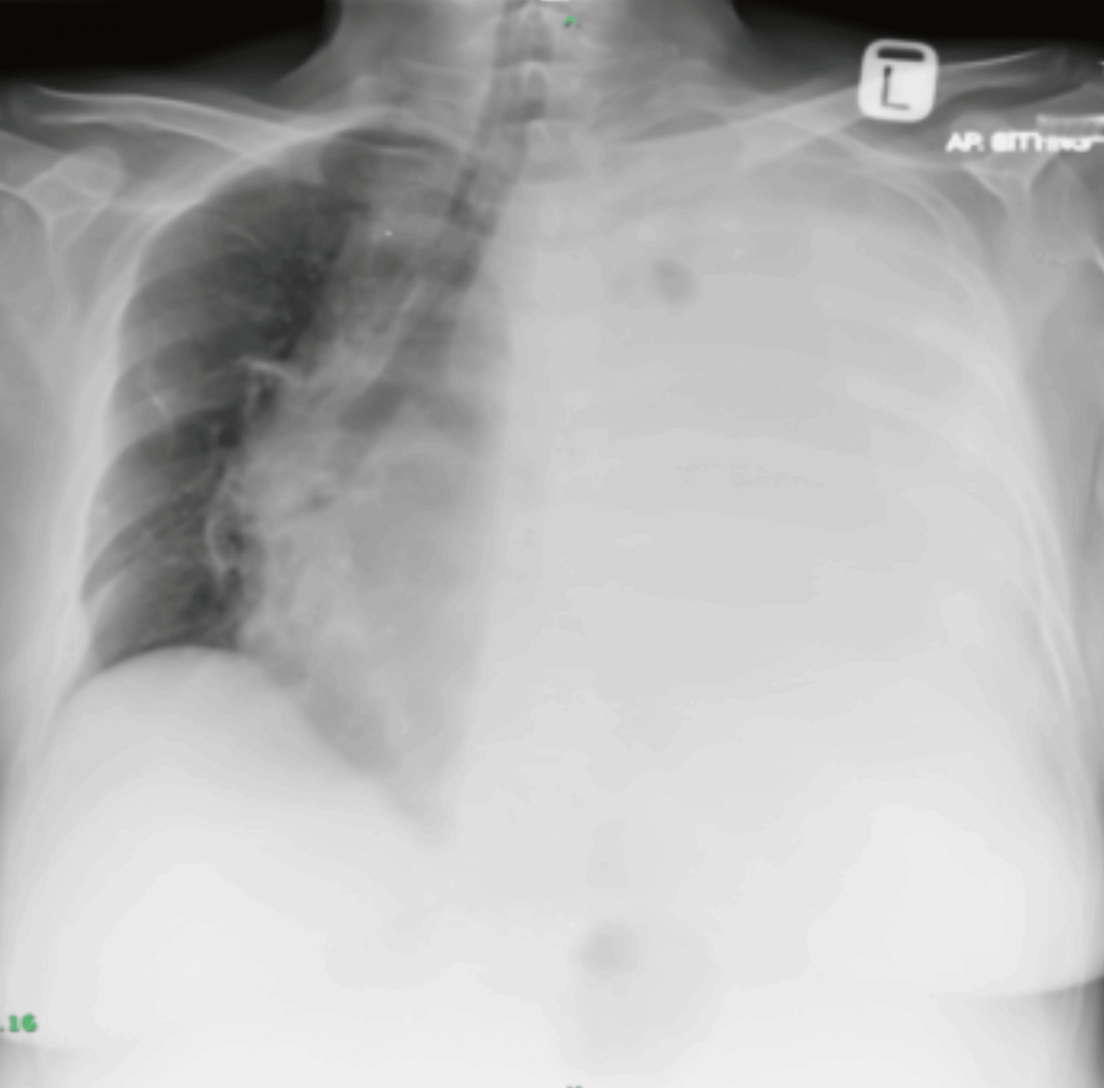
Chest radiograph showing a wiped-out left lung with tracheal and mediastinal shift to the right
